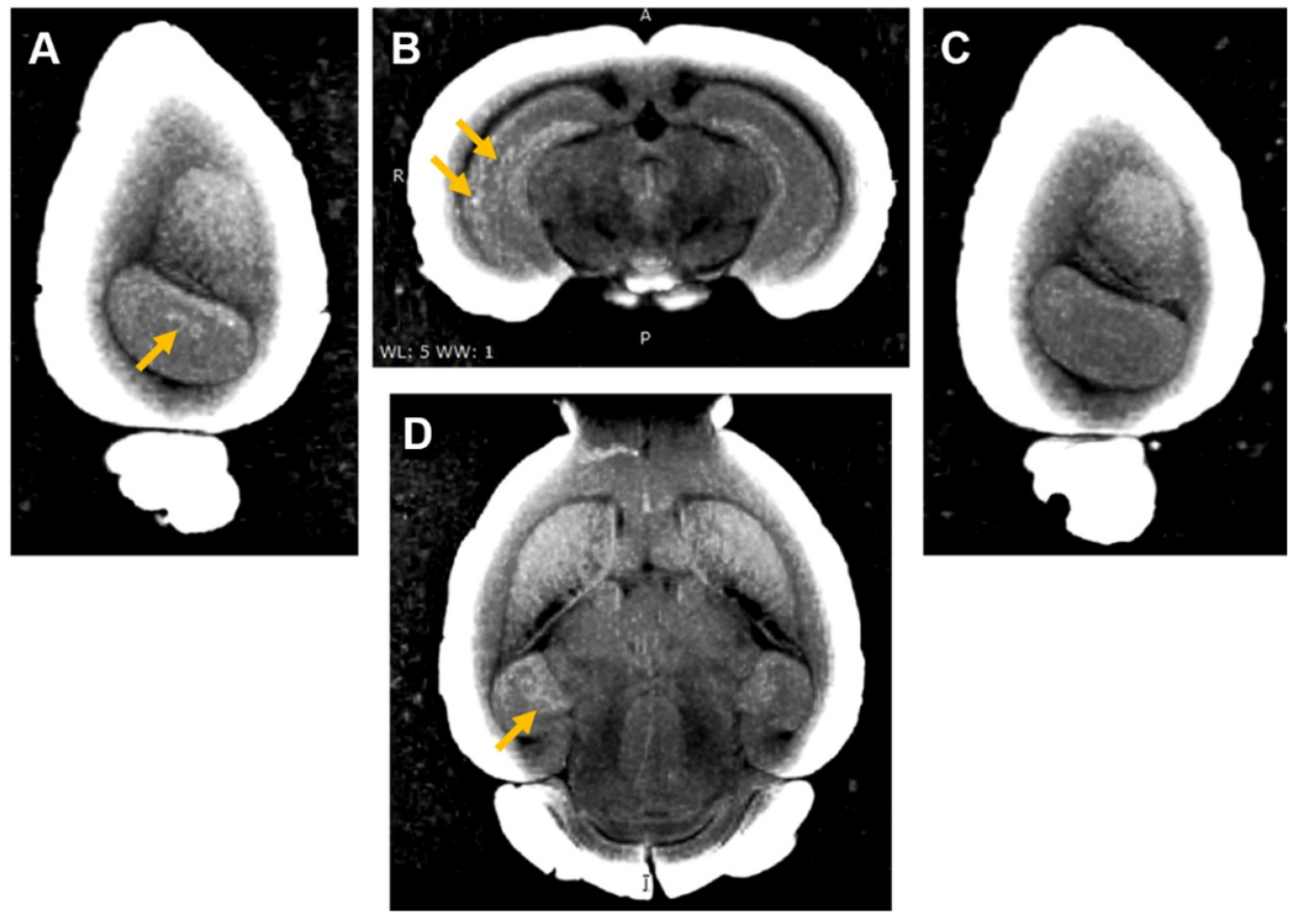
Ex vivo* MRI images of brain with Gd(rhodamine-pip-DO3A) delivery to the left hemisphere. (A) Sagittal, (B) coronal and (D) axial MR views show enhanced T_1_ contrast in the left hippocampus compared to the (C) contralateral side. The localized distribution of the signal (arrows) confirms the targeted delivery of Gd(rhodamine-pip-DO3A). The periphery of the brain slices has a high intensity due to the increased contrast applied to the images to visualise the probe.
